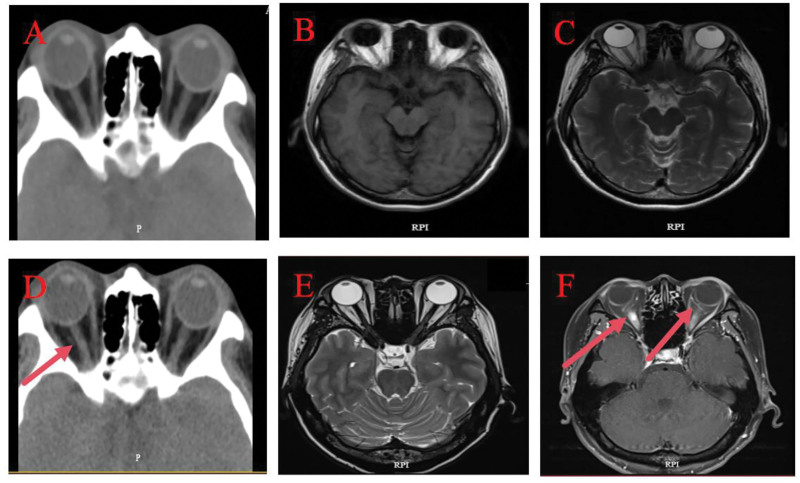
Patient’s initial CT and MRI orbital images. CT = computed tomography, MRI = magnetic resonance imaging.
